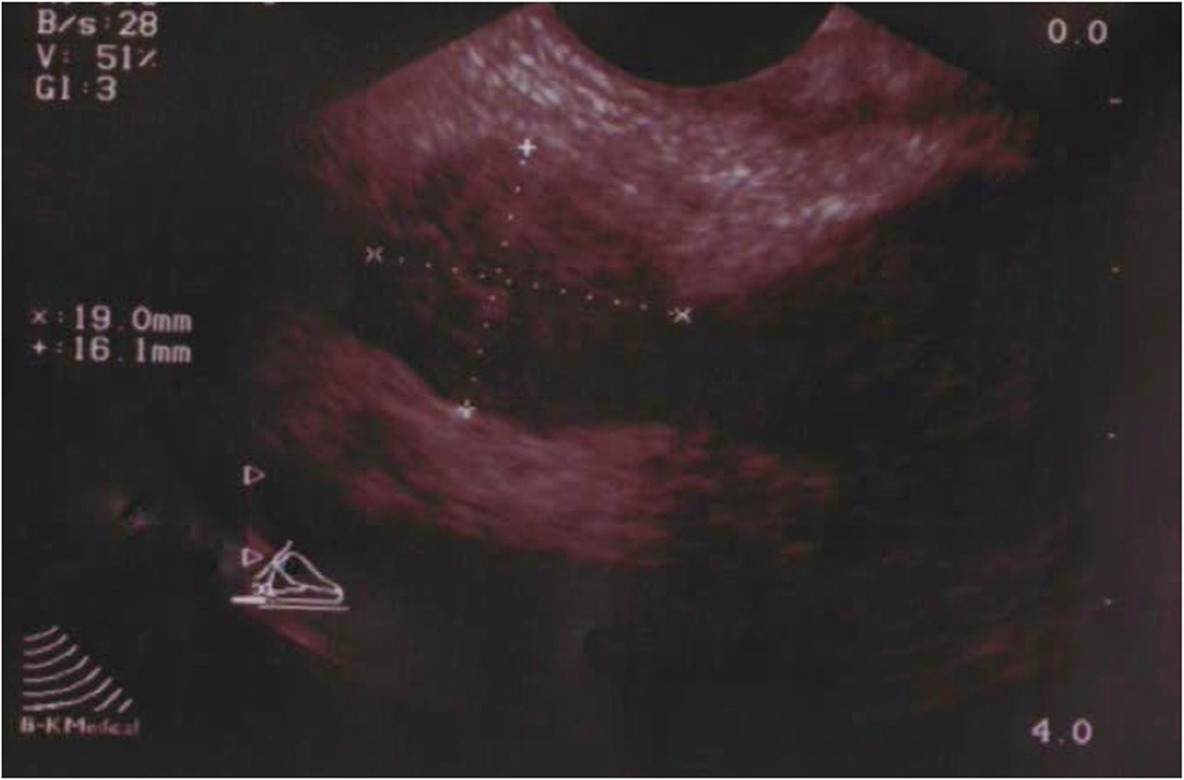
Ultrasound of the right seminal vesicle mass.
